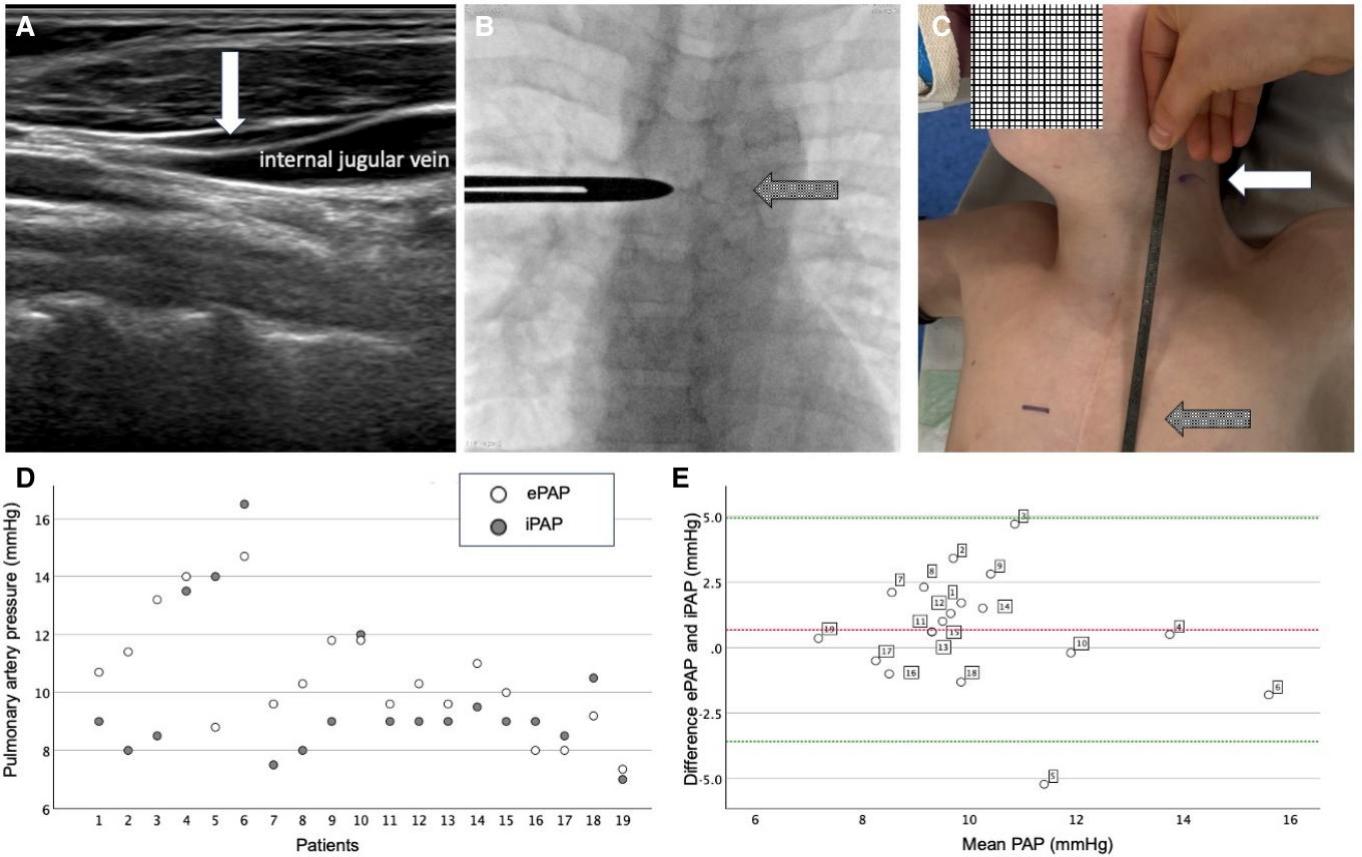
(*A*) The collapse point of the internal jugular vein was localized by ultrasound (white arrow) in an upright position and was marked on the skin. (*B*) At the end of catheterization, the level of the pulmonary arteries (PAs) was located by fluoroscopy and was also marked on the skin (grey arrow). (*C*) The craniocaudal distance between the collapse point of the internal jugular vein and the level of the PAs was measured and used for the estimation of PAP. (*D*) Estimated non-invasive pulmonary artery pressure (ePAP, open circles) and invasive measurements (iPAP, grey circles) of each participant. Note that ePAP and iPAP did not differ > 2 mmHg during the last 10 measurements. (*E*) Bland–Altman plot depicting agreement between ePAP and iPAP. The central red dotted line represents the mean difference (0.67 mmHg) between the iPAP and ePAP measurements while the green dotted lines represent the standard deviation. There was only one outlier (Subject No. 5).
